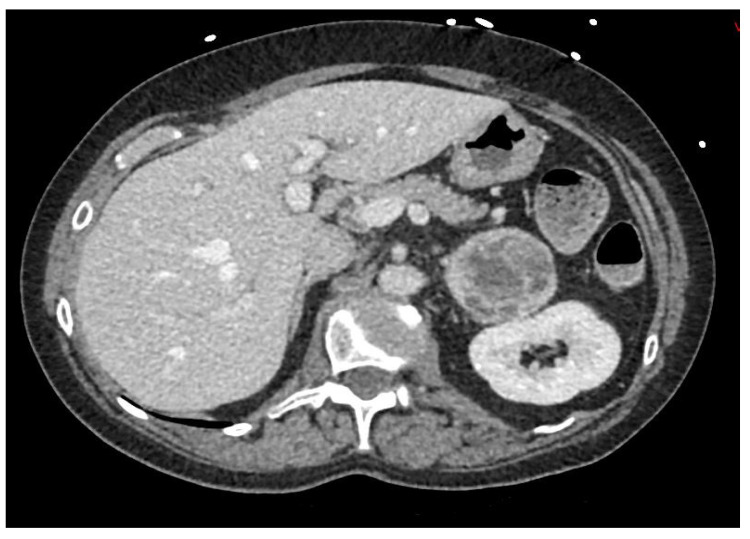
Abdominal CT (DFOV 85 × 37 mm): Pheochromocytoma, left heterogenous adrenal tumor.
